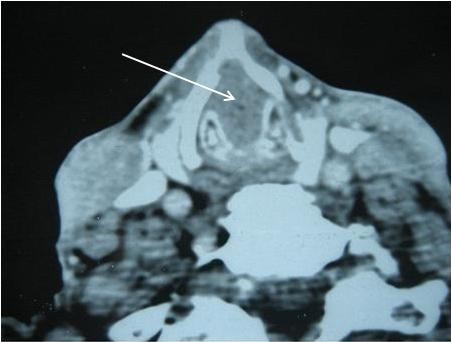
Computed tomography scan showing a subglottic tumor completely obstructing the laryngeal lumen. Arrow shows the subglottic tumor while the glottis area is free of disease (Figure 1).
